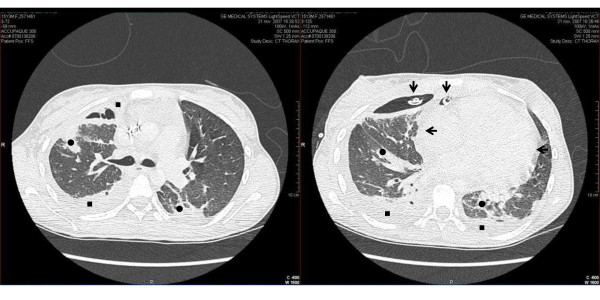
CT scan of the thorax showing bilateral necrotizing pneumonia (black circles) and pleural effusion (black squares) as well as pericardial effusion (leftwards arrows). Note the presence of a chest tube inserted into the pleural cavity (in communication with the lung abscess) and a drainage tube into the pericardial cavity (downwards arrows).
